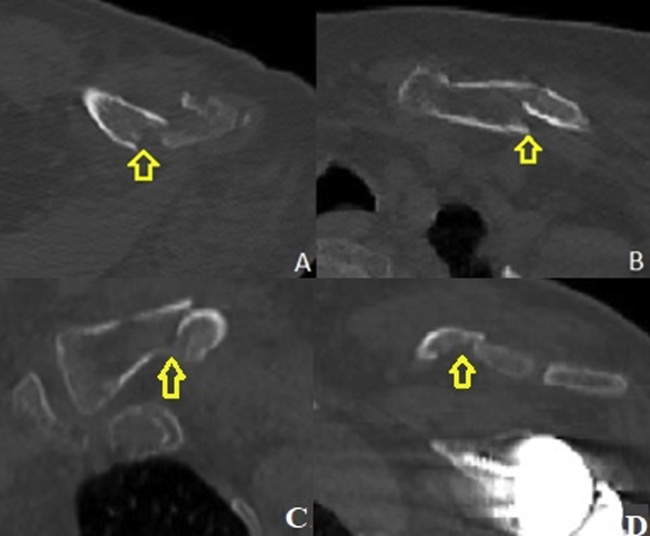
CT scan of the Left shoulder (A) axial cut showing distal displaced clavicle fracture (B) axial cut showing proximal displaced clavicle fracture (C) coronal cut showing proximal displaced clavicle fracture (D) coronal cut showing distal displaced clavicle fracture with intact glenoid component of previous reverse shoulder prosthesis.
